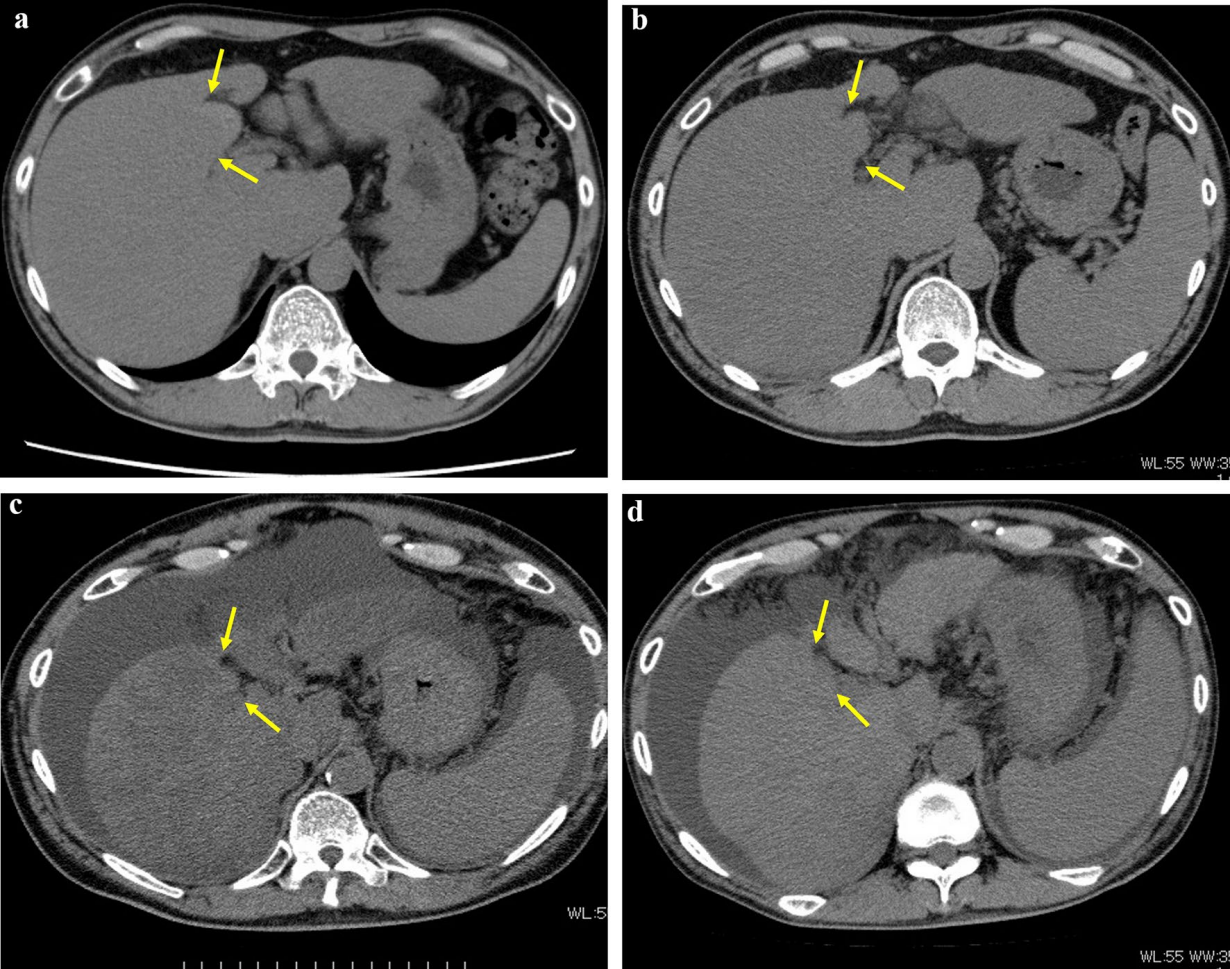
Longitudinal change of gallbladder fossa nodularity (GBFN) in a 47-year-old man with alcoholic liver disease. a Non-contrast CT reveals grade 3 GBFN. ALBI score and modified ALBI grade were − 2.4 and grade 2a. b Non-contrast CT obtained 3 years later reveals less prominent GBFN, but still grade 3 was assigned. ALBI score and modified ALBI grade were − 1.8 and grade 2b. c Non-contrast CT obtained 4 years later (7 years from 4a) reveals markedly shrunk GBFN, for which grade 2 was assigned. Moderate amount of ascites and fatty liver is apparent. ALBI score and modified ALBI grade were − 0.8 and grade 3. d Non-contrast CT obtained one year later (8 years from 4a) reveals further shrinkage of GBFN, for which grade 1 was assigned. Moderate amount of ascites is still present and fatty liver improved. ALBI score and modified ALBI grade were − 1.3 and grade 3, respectively, suggesting substantial deterioration of liver function since 4a. Note prominent splenomegaly and less prominent caudate lobe enlargement as compared to 4a
